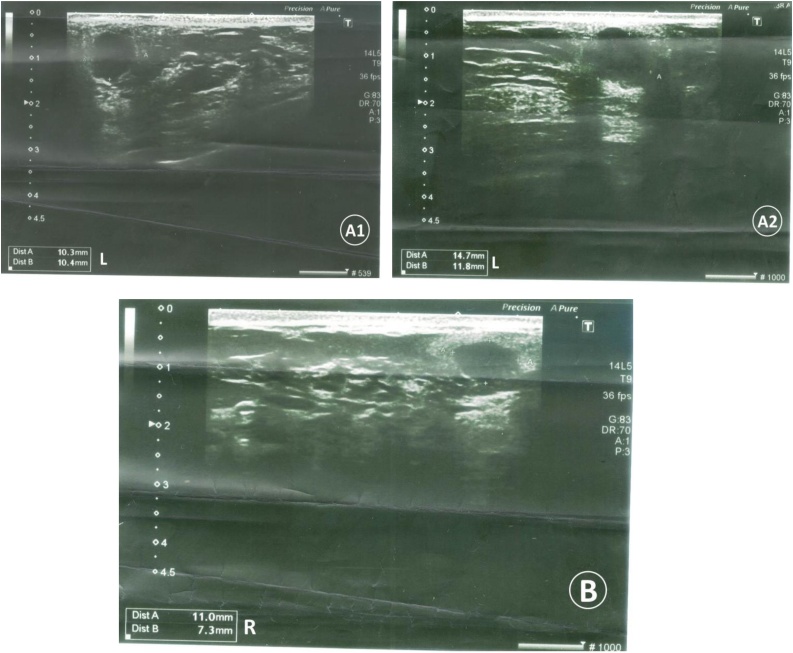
Ultrasound showing (A1, A2) two masses in the left breast (10.3 × 10.4 mm – 14.7 × 11.8 mm) and (B) one in the right breast (11.0 × 7.3 mm) that had irregular margins with central necrotic changes.
